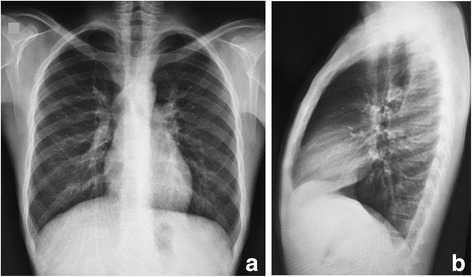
Chest X-ray imaging with no alterations. a Anteroposterior position. b Lateral position
